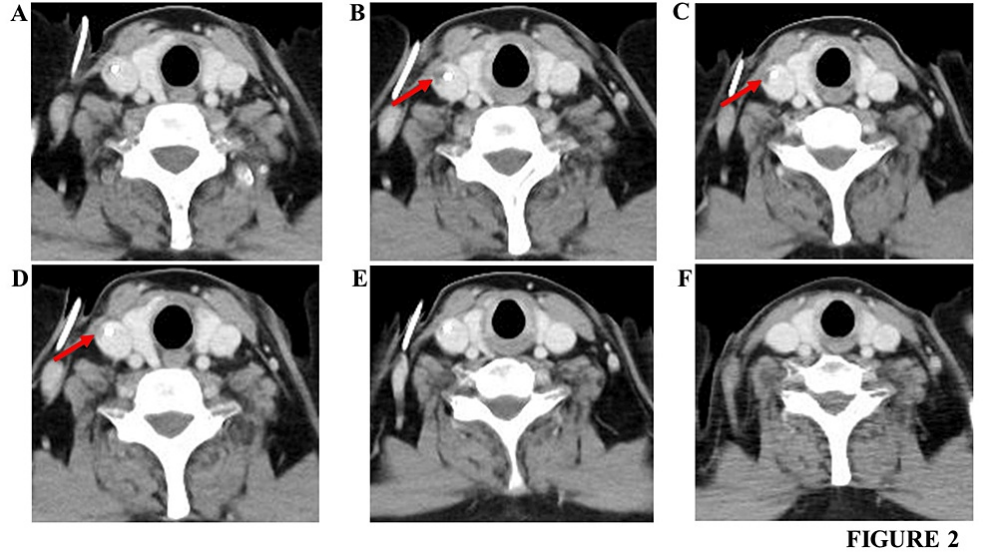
Contrast-enhanced CT findings of the sixth cervical vertebrae in Case 2 (A) CT findings obtained on December 7, 2022. (B) CT findings obtained on January 24, 2023. (C) CT findings obtained on February 2, 2023. (D) CT findings obtained on February 20, 2023. (E) CT findings obtained on March 13, 2023. (F) CT findings obtained on December 4, 2023. Arrows indicate the thrombus in the right internal jugular vein at the CV catheter insertion site in the CV port. CT: computed tomography; CV: central venous
